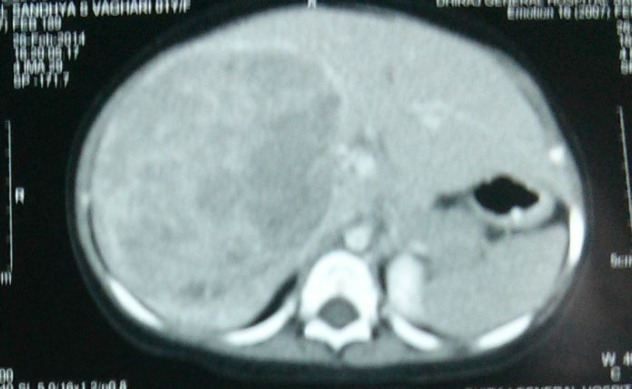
The abdominal CT image showing a heterogeneous enhancing mass in the right lobe of the liver
